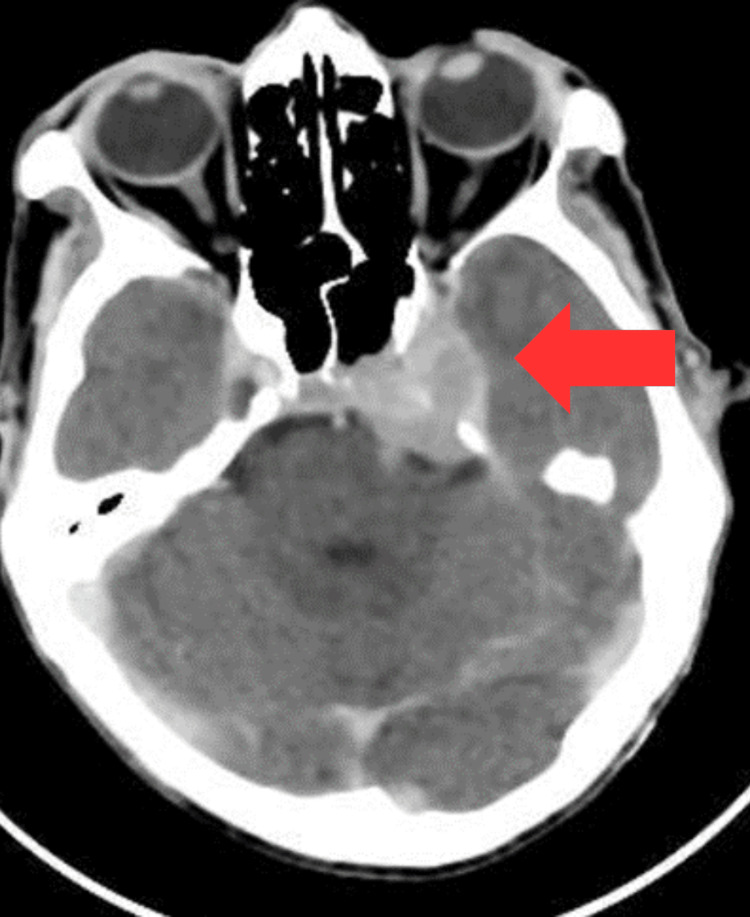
Brain CT post-contrast in mediastinum window demonstrating intracranial extension of the lesion, forming an extra-axial mass in the petroclival region, exerting mass effect on the temporal lobe and obliterating the prepontine cistern CT: computed tomography
